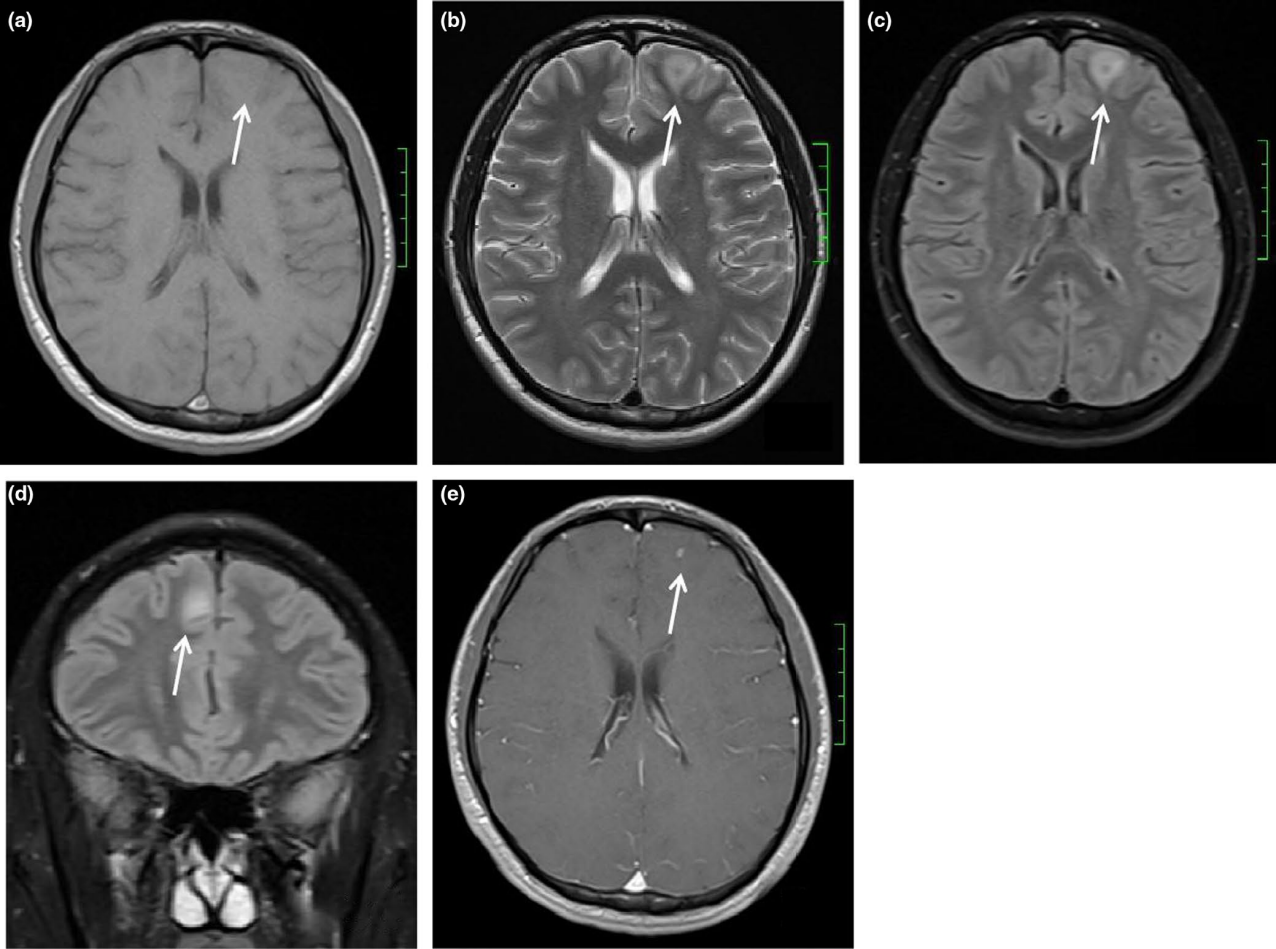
(a–d) Male, 46 years old. (a) T1 axis shows patchy iso‐signal in the left frontal lobe. (b) T2 axis shows patchy long T2 signal in the left frontal lobe. (c, d) Axis and coronal FLAIR show the bilateral frontal lobe lesions with high signal. (e) Axis enhanced shows the bilateral frontal lobe lesions with punctiform enhancement
